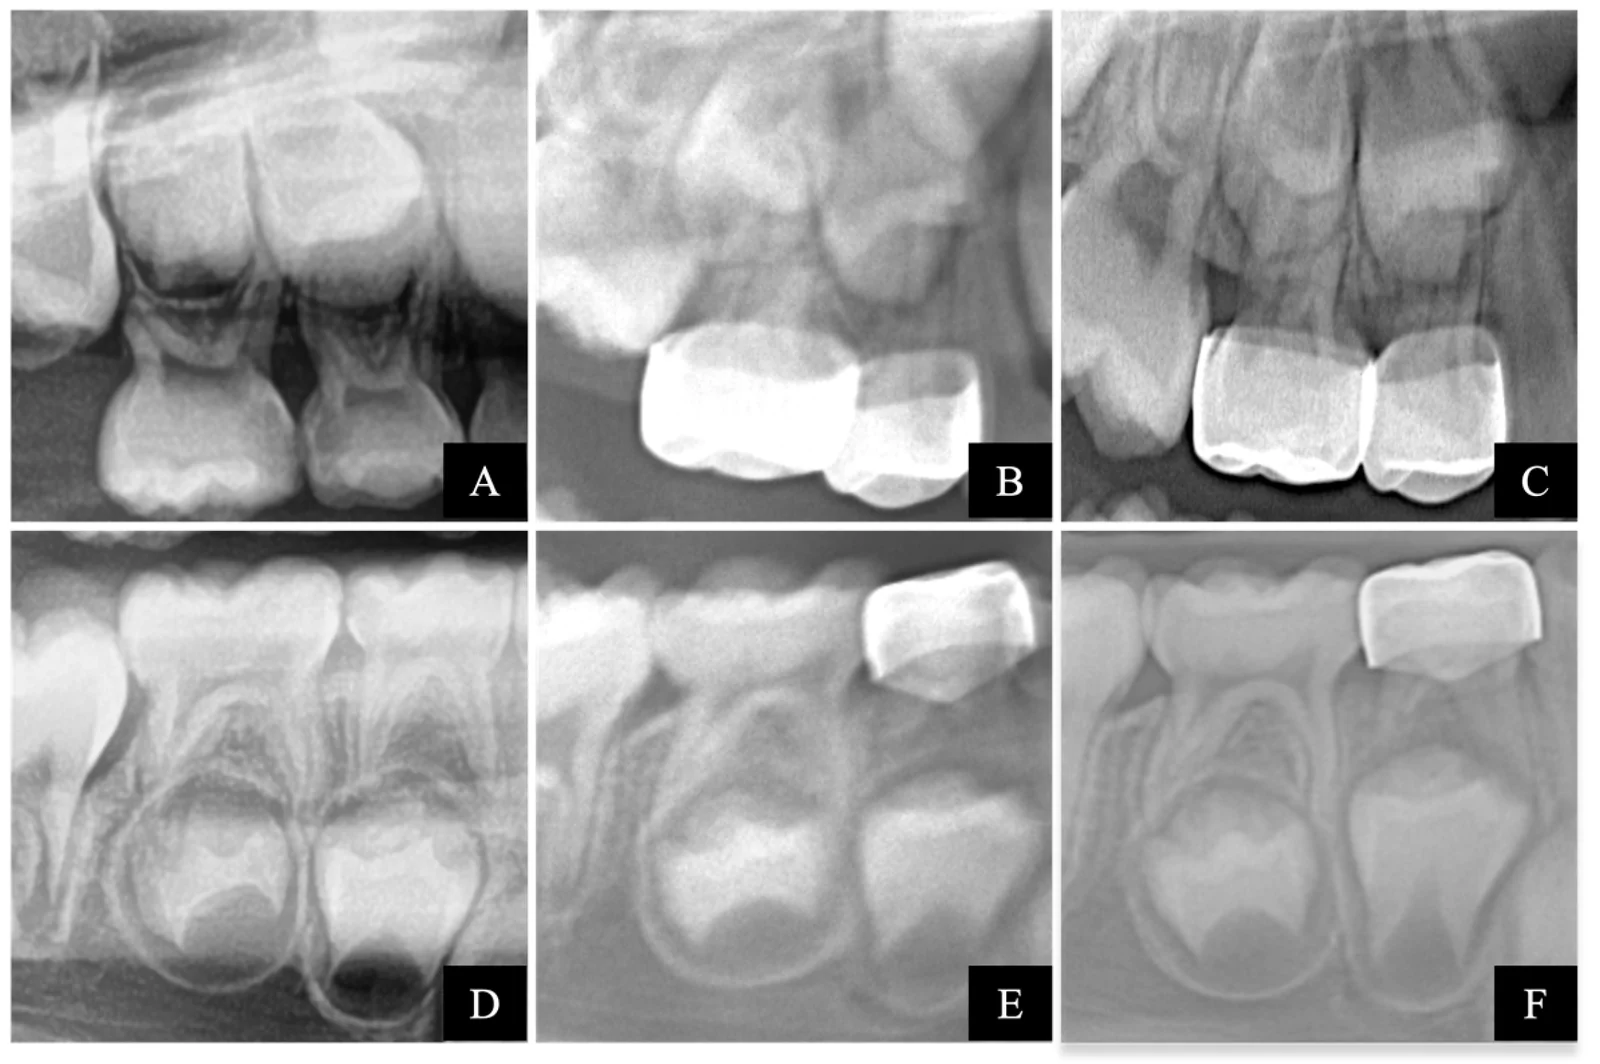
Radiographic evaluation of teeth 54, 55, and 84 in Patient 4. (A–C) Teeth 54 and 55: (A) Preoperative radiograph; (B) 6-month post-treatment radiograph; (C) final follow-up radiograph. (D–F) Tooth 84: (D) Preoperative radiograph; (E) 6-month post-treatment radiograph; (F) final follow-up radiograph.
